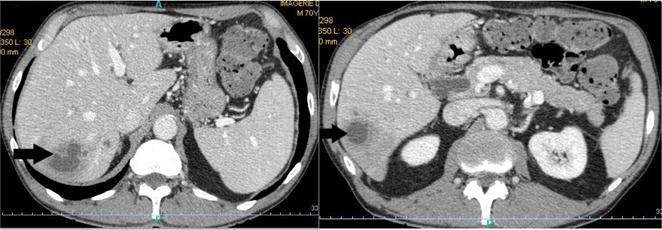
Abdominal CT showing two low density lesions in the right liver (a*rrows*), compatible with the diagnosis of liver abscesses
